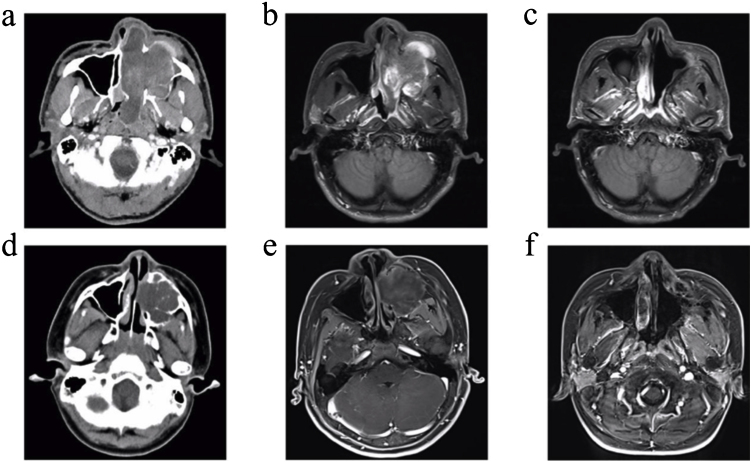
Images of patient 4 and patient 6. (a) Enhanced CT for patient 4 showed a large soft-tissue mass in the left maxillary sinus and nasal cavity that invaded the cheek, nasolacrimal duct dacryocyst area, infratemporal fossa, orbital wall, ethmoid sinus, anterior and posterior nostrils, and partly destroyed the bone of the sinus cavity. (d) Enhanced CT for patient 6 showed a large expansive soft-tissue mass in the left maxillary sinus that invaded the hard palate, nasal cavity, alveolar bone, inferior turbinate, and partly destroyed the bone of the sinus cavity. (b) Enhanced MRI for patient 4 and (e) enhanced MRI for patient 6 showed uneven enhancement of the masses. (c) Enhanced MRI for patient 4 and (f) enhanced MRI for patient 6 revealed no masses in the surgical cavity.
